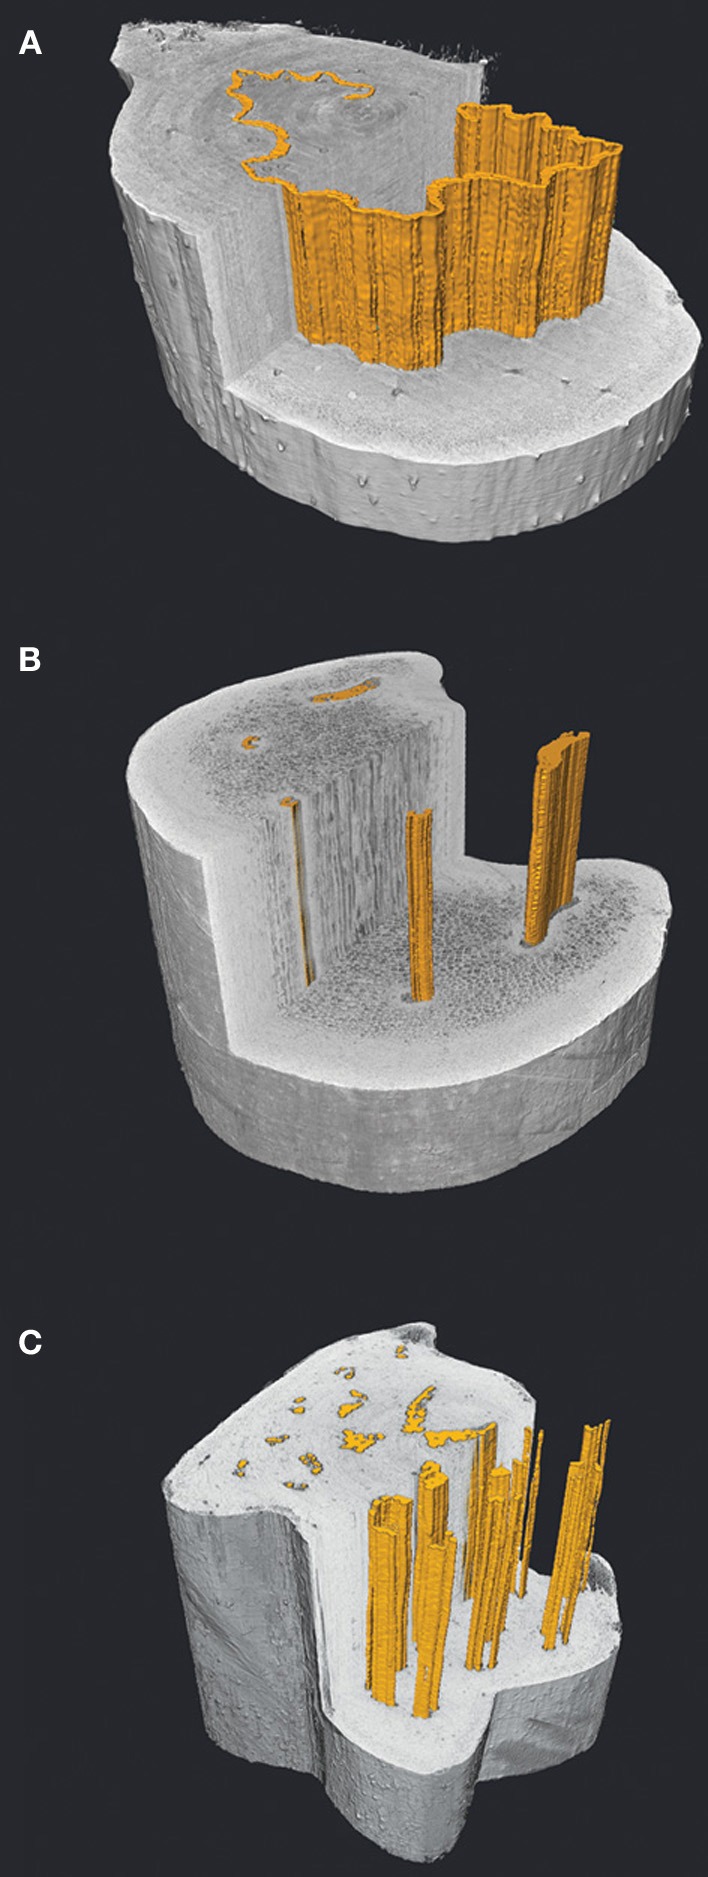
Virtual transverse and longitudinal sections through frond stipes of *Dicksonia antarctica* (A), *Woodwardia fimbriata* (B) and *Polystichum munitum* (C) visualized with high resolution X-ray computed tomography (HRCT). The vascular bundles are highlighted in orange and show a small sample of the diversity of stelar patterns in ferns, with the continuous band of xylem in *D. antarctica*
